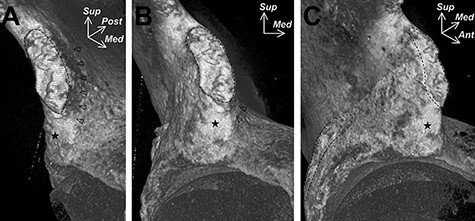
An image derived from micro-computed tomography indicating the shallow groove of the anteromedial surface of the AIIS (indicated by use of arrowheads) where the iliocapsularis was suggested to arise from. The dashed lines correspond roughly to the superior portion of the AIIS and anterolateral wall of the ilium. The star indicates the smooth impression at the inferior portion of the AIIS. Ant= anterior, Med= Medial, Post = posterior, Sup = superior. Image describes the anteromedial (A), anterior (B) and anterolateral (C) aspects of the right hip. Image retrieved from Tsutsumi *et al*. (2019) [16].
